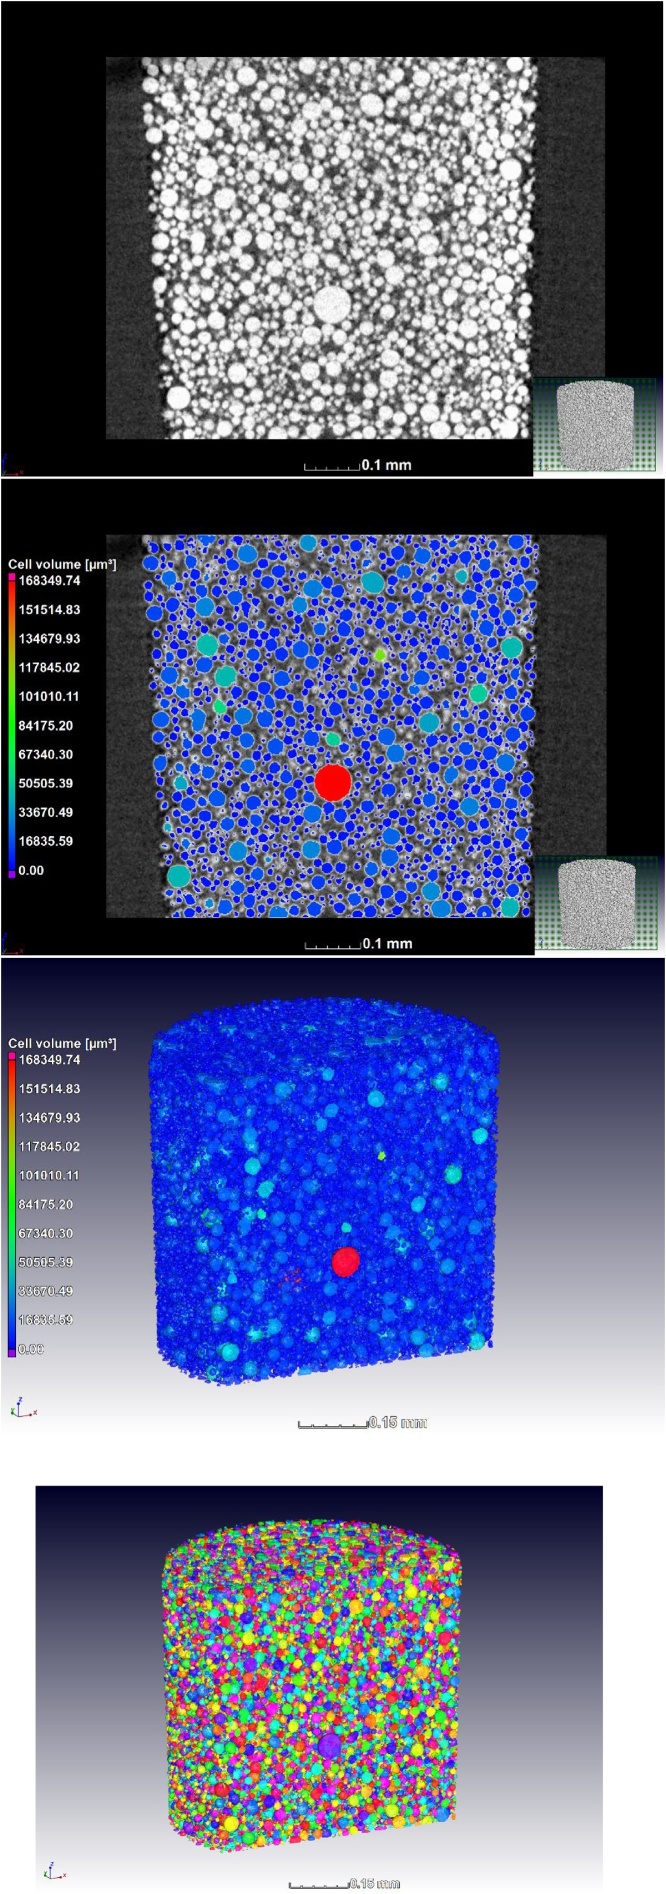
Submicron CT scan of DMLS powder (<40 μm specification). This series shows the slice image without and with analysis, a 3D view of the analysis for size, and a 3D view of the uncoded particles – colours a varied between adjacent particles to highlight the large number of particles.
